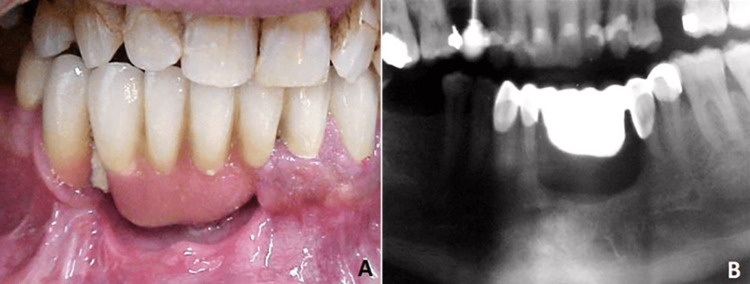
A: Intraoral photograph of the patient showing the removable prosthesis placed three months postoperatively. B: Orthopantomogram of the patient three months postoperatively.
